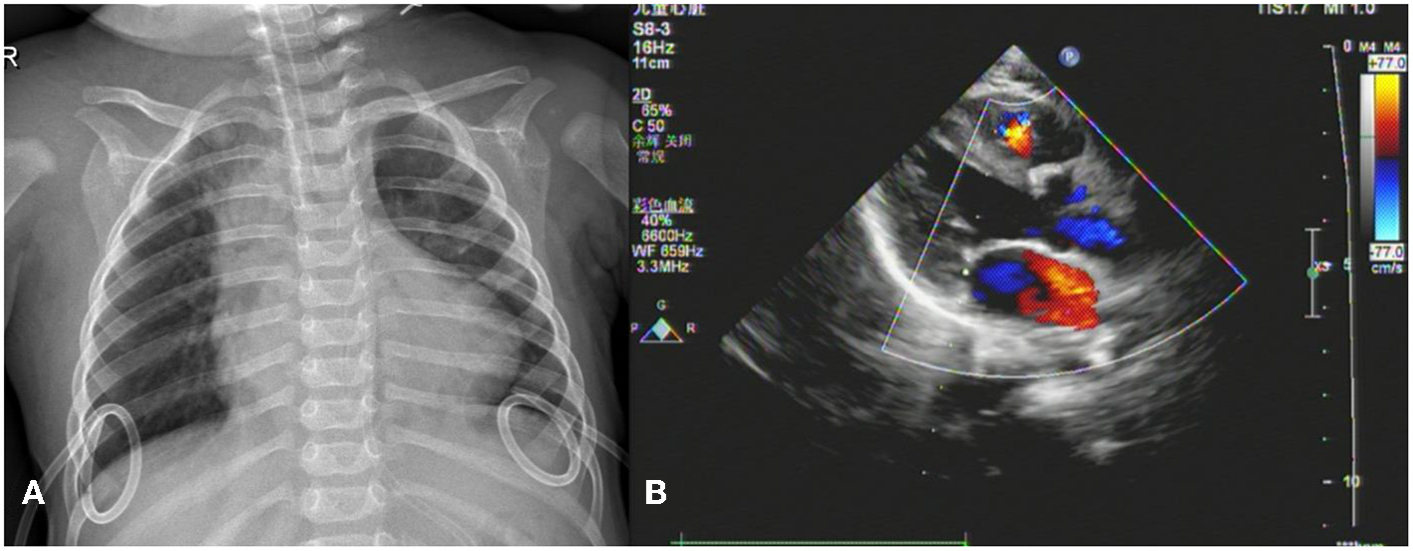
Imaging findings. (A) Chest X-ray shows bilateral drainage tubes and no obvious signs of pleural effusion. (B) No signs of pericardial tamponade or pericardial effusion on echocardiogram in left ventricular long-axis view.
